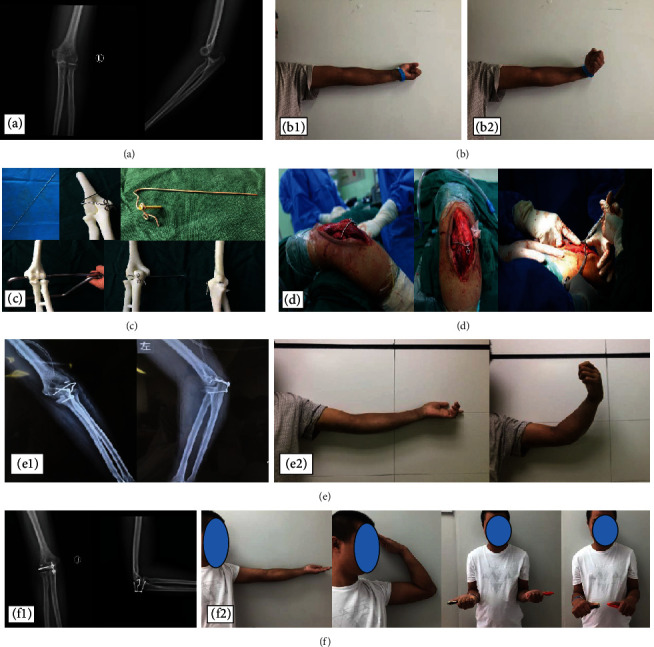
(a) X-ray on admission of a 28-year-old man showing a dislocated left elbow joint. (b-1) Extension function of the elbow. (b-2) Flexion function of the elbow. (c) Individualized design of the intra-articular stabilization device based on the 3D model of the elbow joint injury and repair. (d) Placement of the intra-articular stabilization device. (e-1) X-ray films immediately after placement. (e-2) Active flexion and extension five days after surgery. (f-1) X-ray films two months after placement show that the elbow joint alignment is normal, and the device has not become loose, fallen off, or broken. (f-2) Elbow extension, elbow flexion, forearm supination, and forearm supination functions two months after placement.
